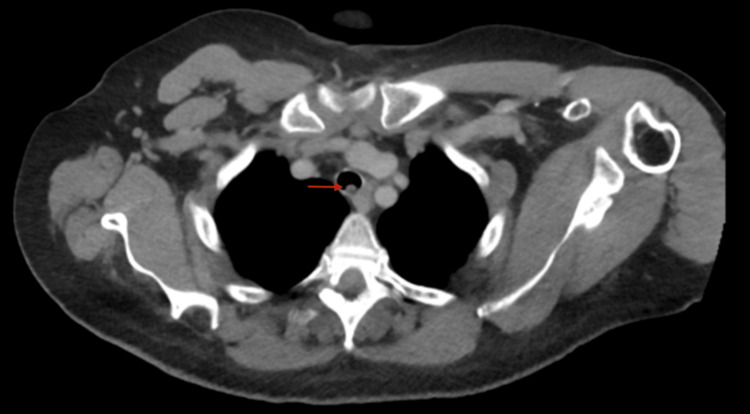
Axial view of the patient's noncontrast CT chest imaging. A metastatic tracheal lesion (red arrow) is demonstrated. The left mainstem bronchus lesion was not detected CT: computed tomography
